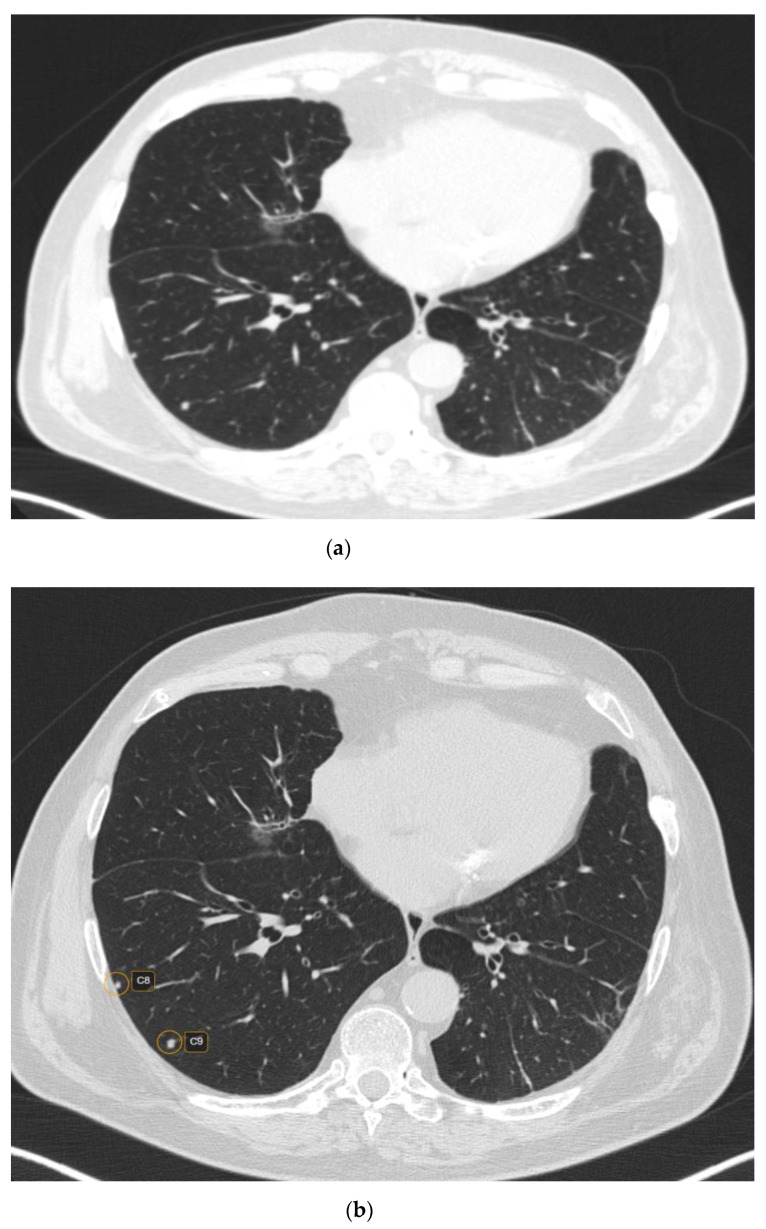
CT scan (lung window) showing raw CT images (a) versus marked CT images using CAD (b).
